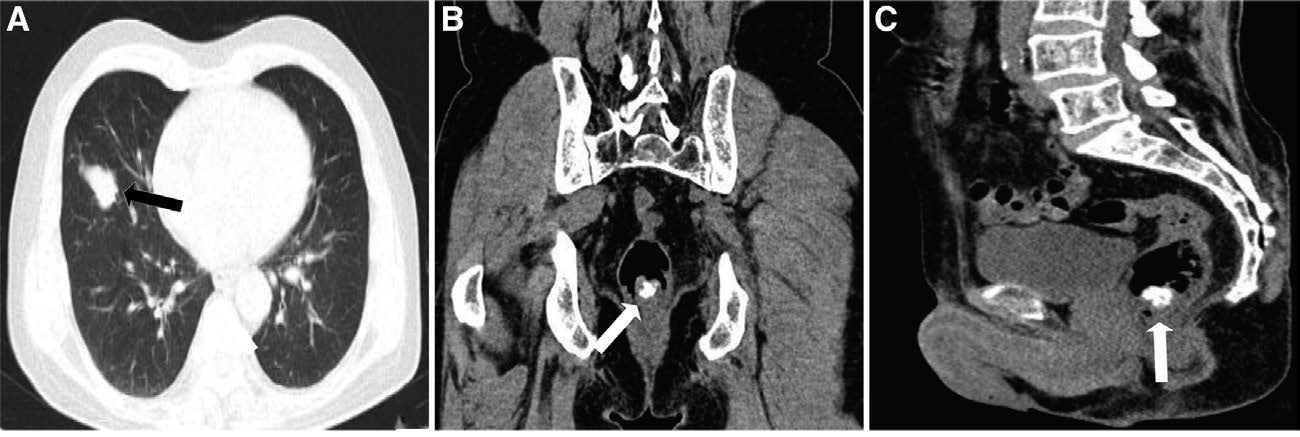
Radiological findings. (A) Thoracic CT indicated irregular nodular soft tissue density shadow in the middle lobe of the right lung (black arrow). (B, C) Axial view and sagittal view of abdominal CT revealed a rectal mass with irregular calcification near the anus (white arrow). CT = computed tomography.
